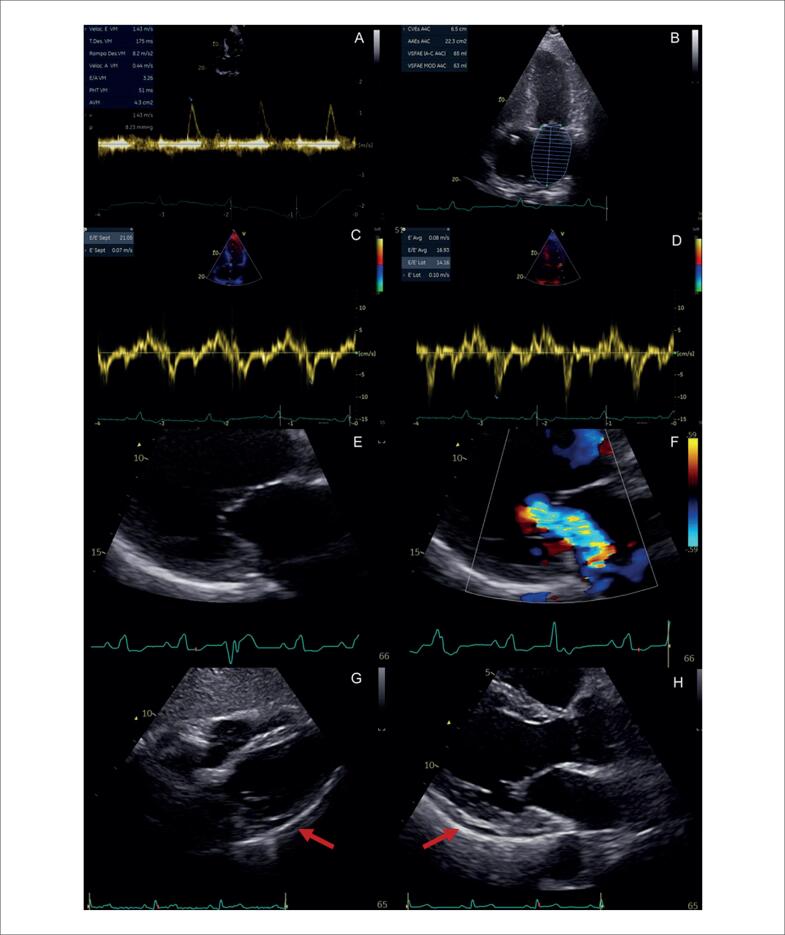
Alterações menos específicas de acometimento cardíaco por sarcoidose ao ecocardiograma transtorácico. Em A, B, C e D, é caracterizada disfunção diastólica de grau restritivo (relação E/A = 3,2, volume indexado do átrio esquerdo de 39 mL/m² e relação E/e’ = 16,9). A: Doppler espectral do influxo mitral. B: Volume do átrio esquerdo avaliado pelo método de Simpson, na janela apical 4-câmaras. C: Doppler tecidual do anel mitral medial. D: Doppler tecidual do anel mitral lateral. Em E e F, evidencia-se valva mitral e insuficiência na janela paraesternal de eixo longo focada na valva mitral. E: Modo bidimensional evidenciando falha de coaptação associada a tracionamento apical das cúspides e dilatação do anel mitral. F: Análise pelo Doppler colorido evidenciando jato de regurgitação mitral importante. G e H: observa-se derrame pericárdico localizado posteriormente, de grau discreto (setas), nas janelas subcostal de quatro câmaras (G) e paraesternal de eixo longo (H).
